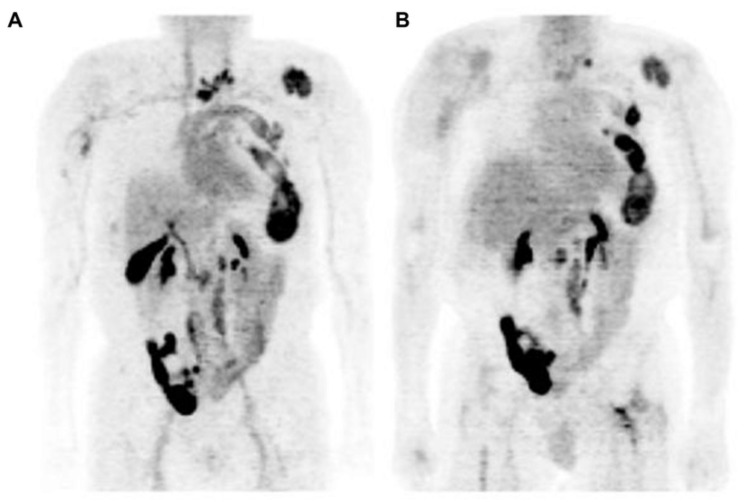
Comparison of [^18^F]FDHT (left (A)) and [^18^F]FDG (right (B)) PET in maximum-intensity projection (MIP) views with widespread bone metastases involving cervical spine, left ribs, and left para-aortic lymph nodes: physiological urinary activity in a right lower abdominal quadrant urinary diversion is also seen [28]. Note: [28] © by the Society of Nuclear Medicine and Molecular Imaging, Inc. (Reston, VA, USA).
